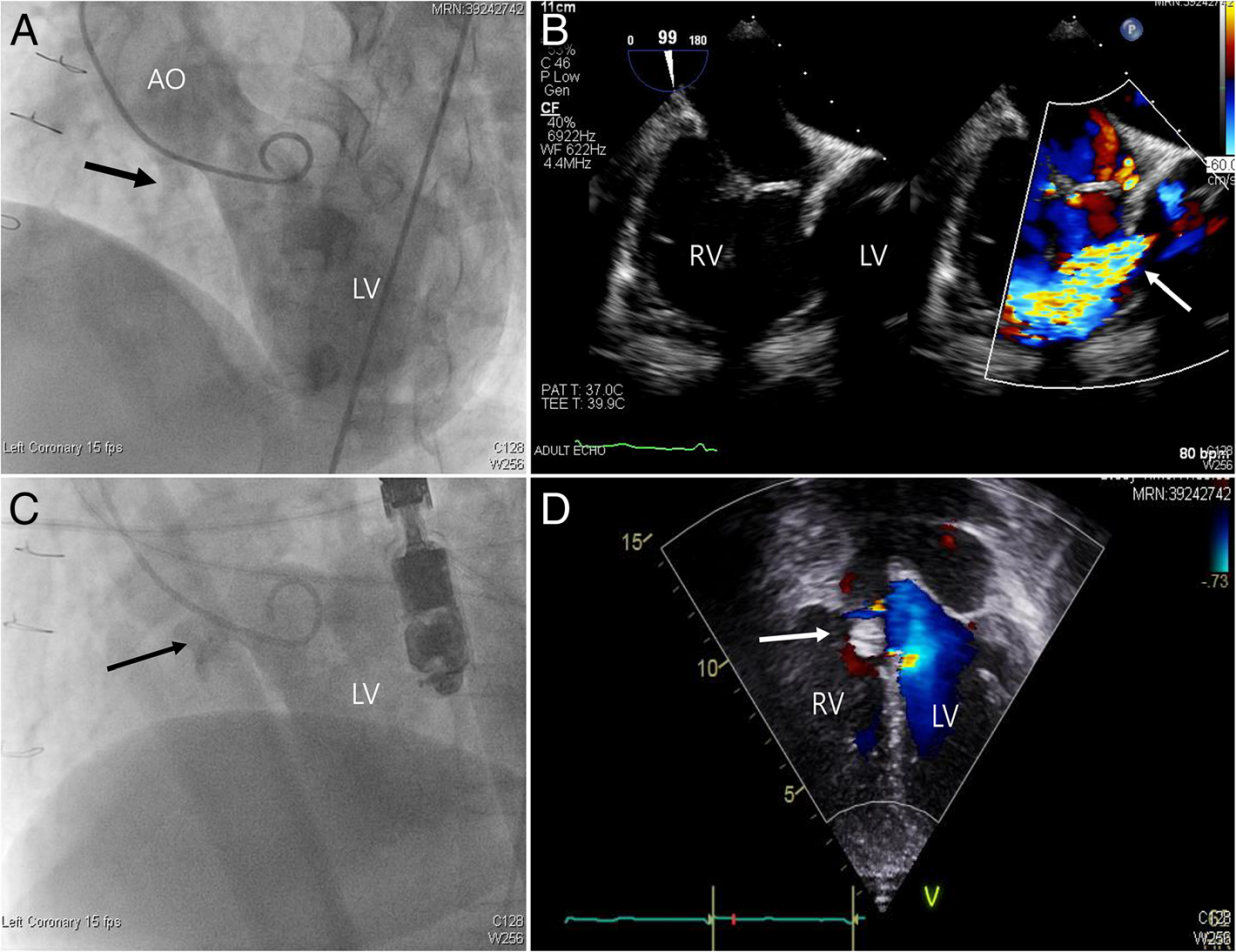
Device closure of residual postopertive VSD with Coccoon VSD occlude device. a LV angiography showed residual VSD (black arrow). b transesophageal echocardiography and color Doppler revealed residual VSD (white arrow) with significant amount. c LV angiography showed successful implantation of the Cocoon device (black arrow) with no significant leakage. d transthoracic echocardiography showed successful device closure after the procedure (white arrow). LV, left ventricle, AO, aorta, RV, right ventricle, VSD, ventricular septal defect
